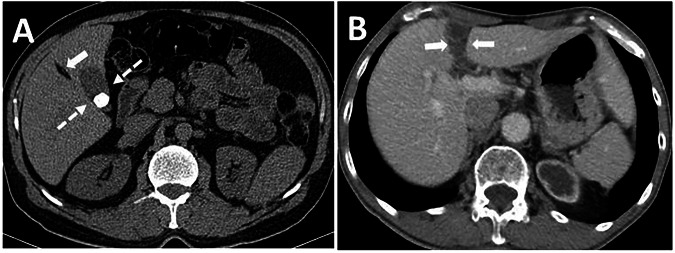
A The unenhanced axial plane CT image shows the right-sided LTH (arrow) and the gallbladder located on the left of the LTH (dashed arrows). Segment IV was atrophic (not shown). B Axial plane contrast-enhanced CT image shows the gallbladder (arrows), which is located in the FL
